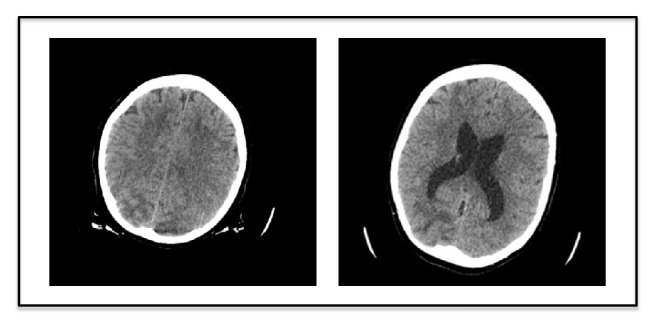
Brain CT-scan showing widespread bilateral asymmetrical hypodensities in the cortex and subcortical regions.
